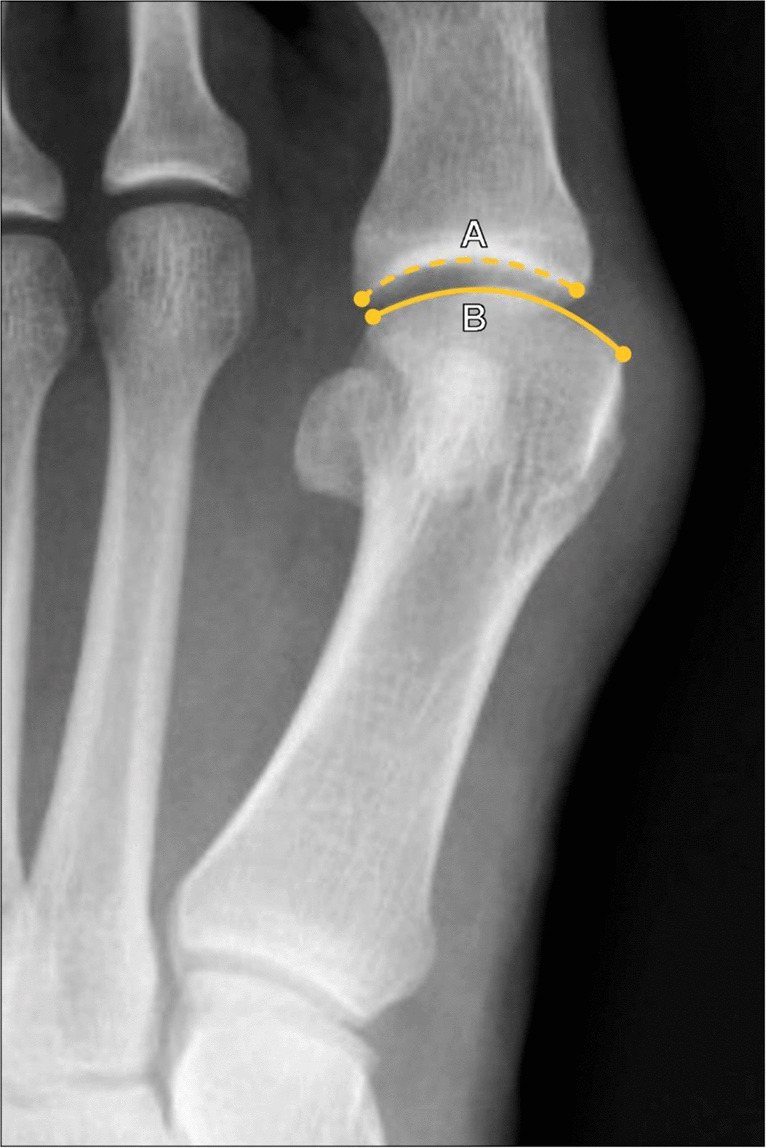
Measurement of Congruence Index on DP radiograph of the foot. The measurement indicates the degree of metatarsal head coverage by the proximal phalangeal base. To calculate the ratio, the portion of the first metatarsal head covered by the proximal phalanx (A, dashed line) is divided by the total length of the first metatarsal head articular surface (B, solid line) and multiplied by 100% to give a percentage. Normal values are > 76.5%, and lower values indicate greater incongruency
